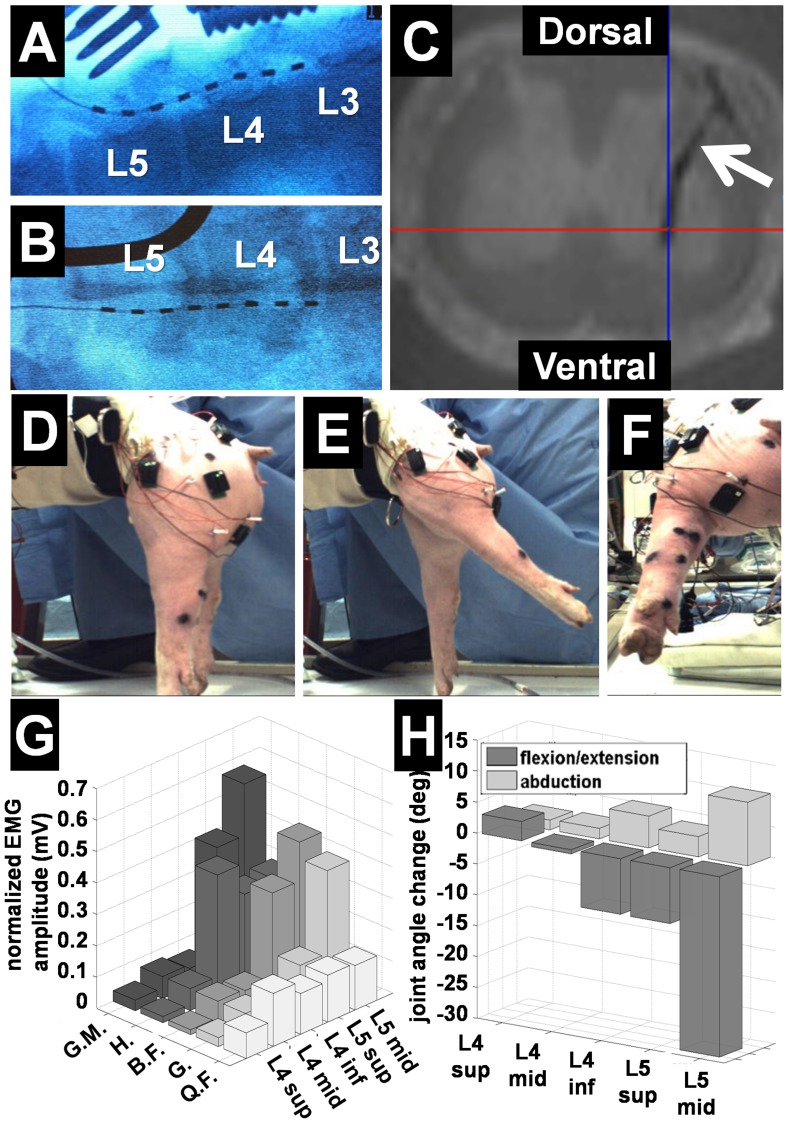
Targeting analysis and functional outcomes. (A) Epidural electrode placement confirmed by lateral intra-operative fluoroscopy; (B) Posterior-anterior intra-operative fluoroscopy; (C) *Ex-vivo* axial MRI of spinal cord showing ISMS electrode tract (arrow); (D) Experimental setup for kinematic analysis; (E–F) Typical hip extension evoked by ISMS at L5-segment; (G) Normalized intramuscular EMG amplitude as a function of spinal cord segment evoked by ISMS at 300 µA (n = 3); (H) Kinematic analysis of joint angle change evoked by ISMS as a function of spinal cord segment (n = 3). Abbreviations: G.M. = Gluteus medius, H = Hamstrings, B.F. = Biceps femoris, G = Gastrocnemius, Q.F. = quadriceps femoris.
